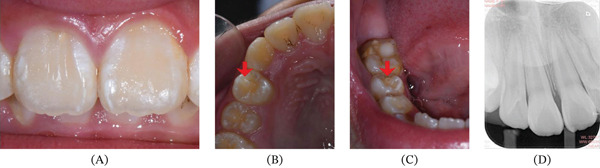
Clinical photographs and radiograph of 18‐month follow‐up. (A) 18‐month follow‐up. (B) #14 after multiple periodic grinding of abnormal central cusp (red arrow). (C) #45 after multiple periodic grinding of abnormal central cusp (red arrow). (D) 18‐month follow‐up periapical X‐ray showing well‐developed root.
